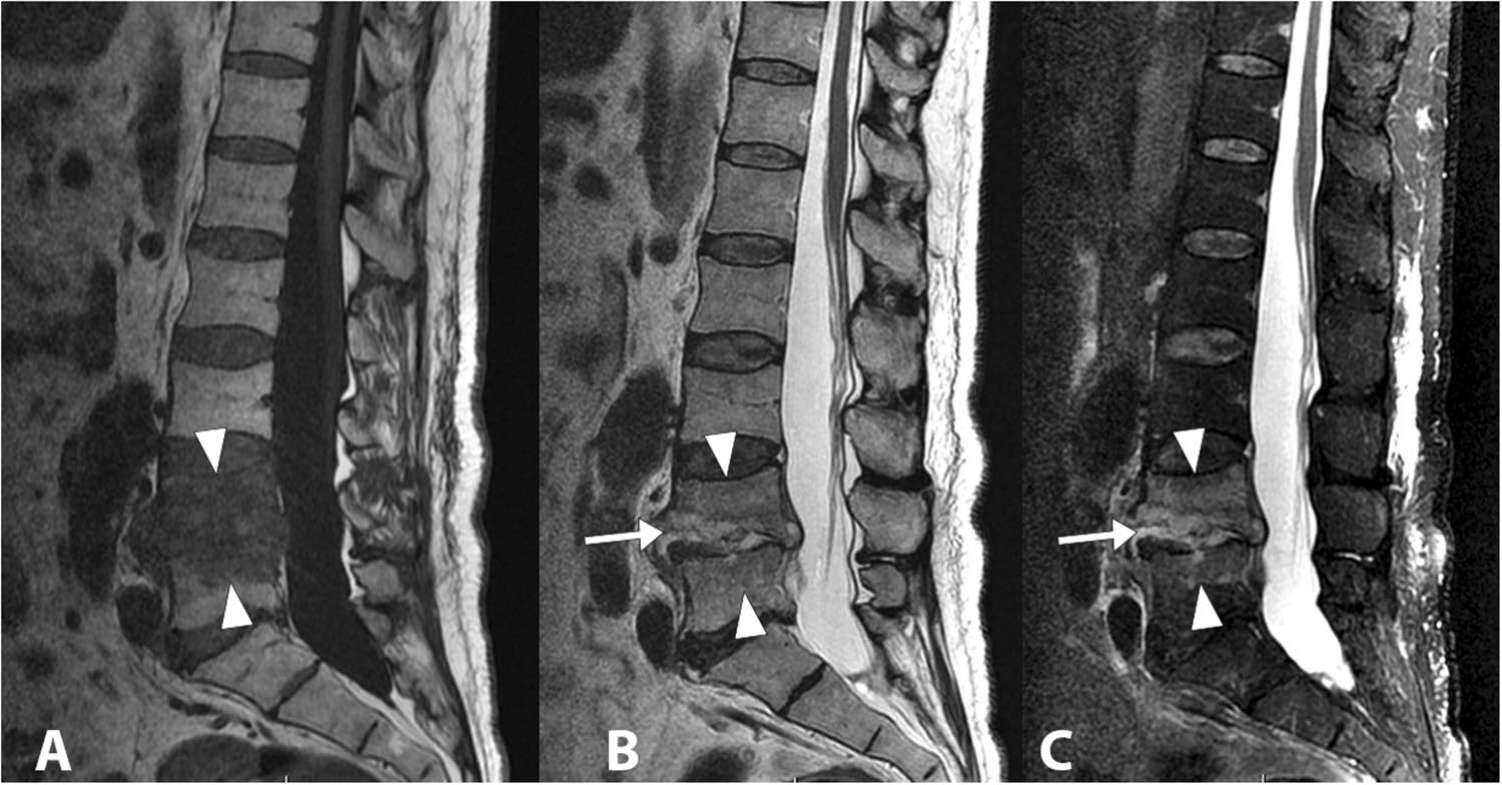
Lumbar (L4-L5) spondylodiscitis caused by Staphylococcus aureus. The whole vertebral body of L4 and the upper portion of L5-vertebral body show an altered signal intensity in the T1-weighted image (a), in the T2-weighted image (b) and in the fat saturated T2-weighted image (c) (arrowheads). The L4–L5 disc is involved and it appears thinned, with an increased signal intensity in the T2-weighted and fat saturated T2-weighted images (arrows)
